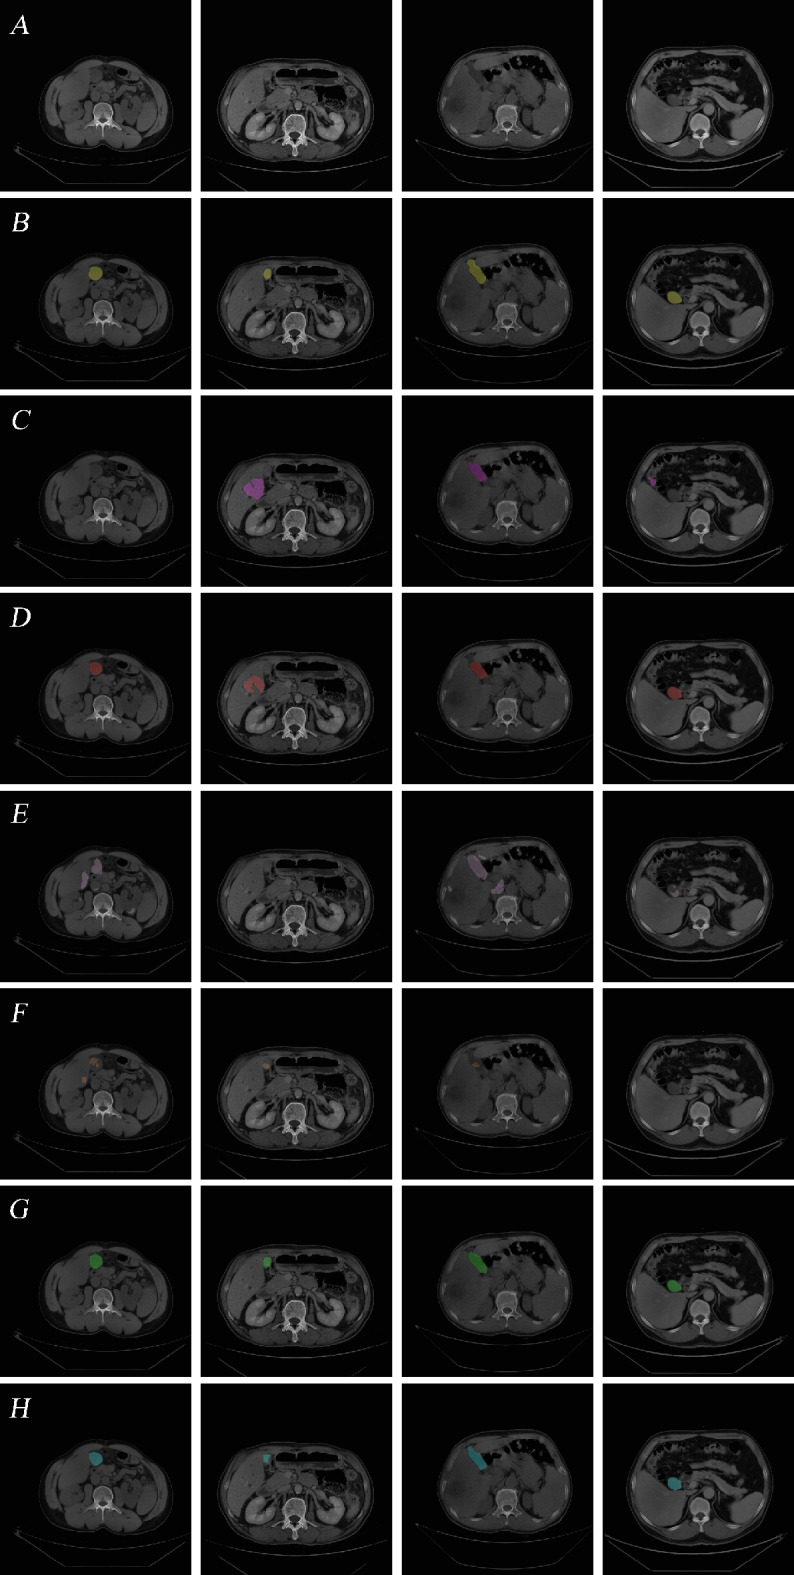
CT image and gallbladder segmentation by the six models (A) Original CT image. (B) Expert-delineated GT. (C) Segmentation by U-Net. (D) Segmentation by Attention U-Net. (E) Segmentation by TransUNet. (F) Segmentation by Swin-Unet (G) Segmentation by MSAU-Net V1. (H) Segmentation by MSAU-Net V2
